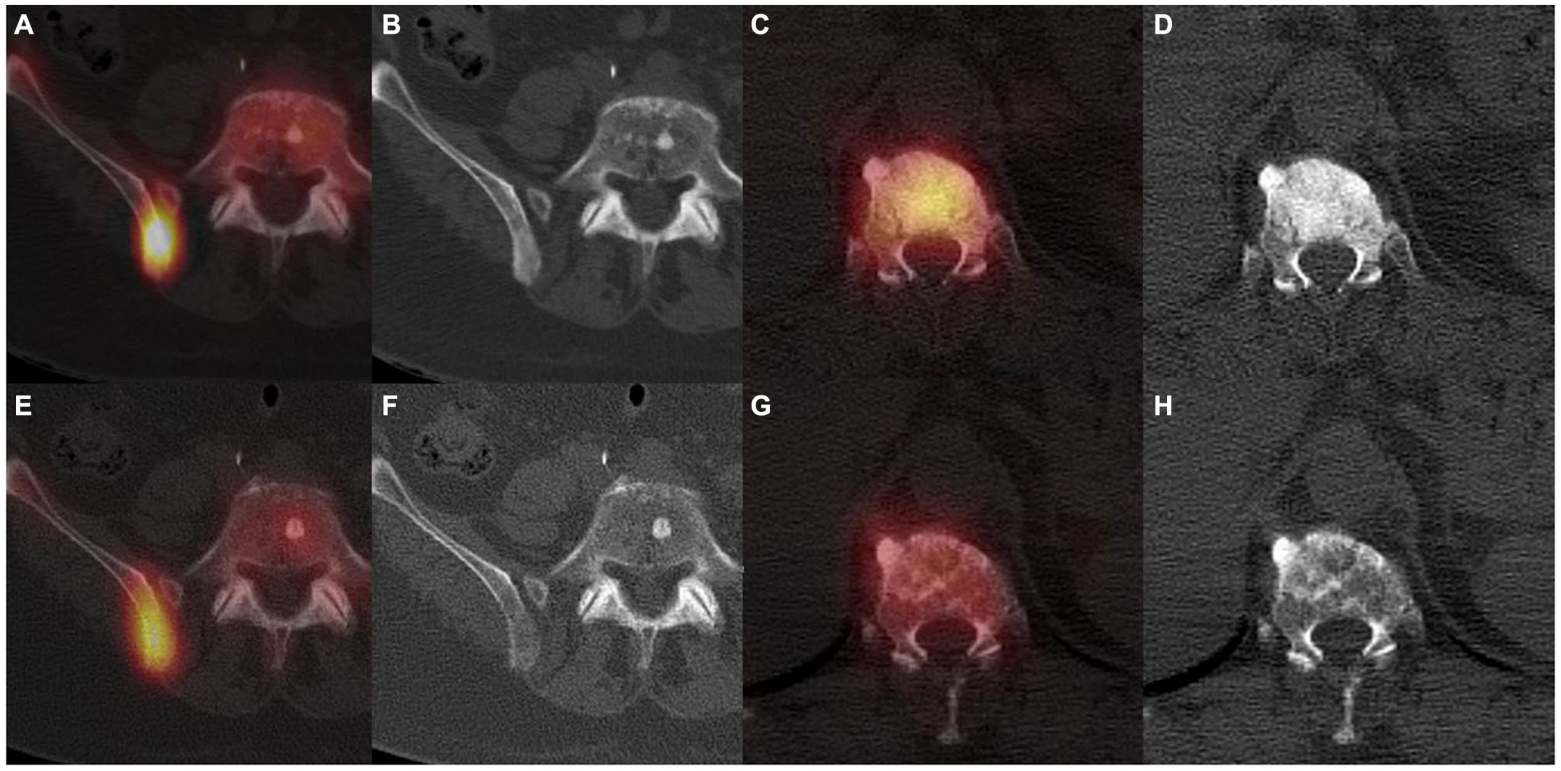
^99m^Tc-HMDP SPECT/CT in a first patient with a bone metastatic prostate adenocarcinoma under first generation hormonotherapy for 2 years, showing a right posterior iliac sclerotic bone metastasis with a high uptake (A,B). Routine follow-up 6 months later showed shading of the iliac metastasis and less intense uptake (E,F), linked to a progression confirmed by a rising PSA and subsequent imaging. ^99m^Tc-HMDP SPECT/CT in a second patient with a bone metastatic breast invasive lobular carcinoma, displaying a vertebral sclerotic metastasis with a high uptake at initial staging (C,D). Routine follow-up 12 months later showed a shadowing of the metastasis and a less intense uptake (G,H), indicative of a therapeutic response confirmed by clinical evolution and subsequent imaging.
